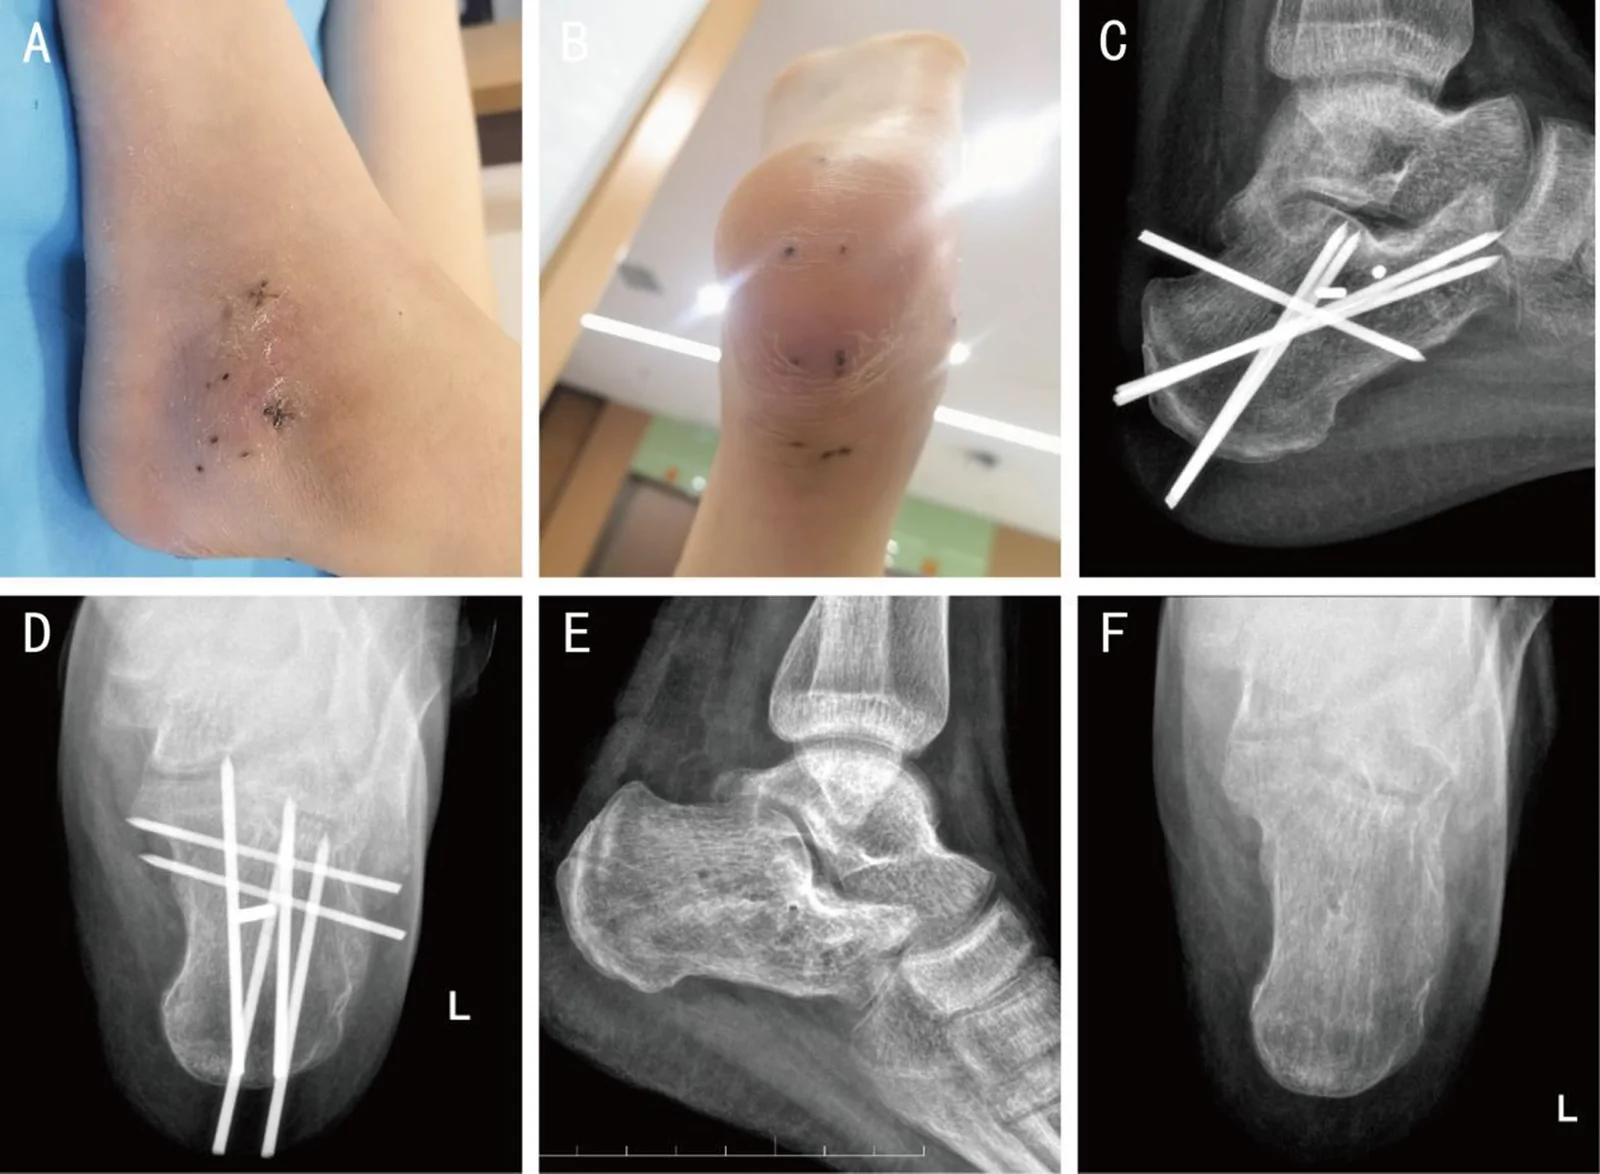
Postoperative follow-up. (A) Healed wound, lateral view; (B) Healed wound, posterior view; (C) Lateral radiograph before Kirschner wires removal; (D) Axial radiograph before Kirschner wires removal; (E) Lateral radiograph after Kirschner wires removal; (F) Axial radiograph after Kirschner wires removal.
